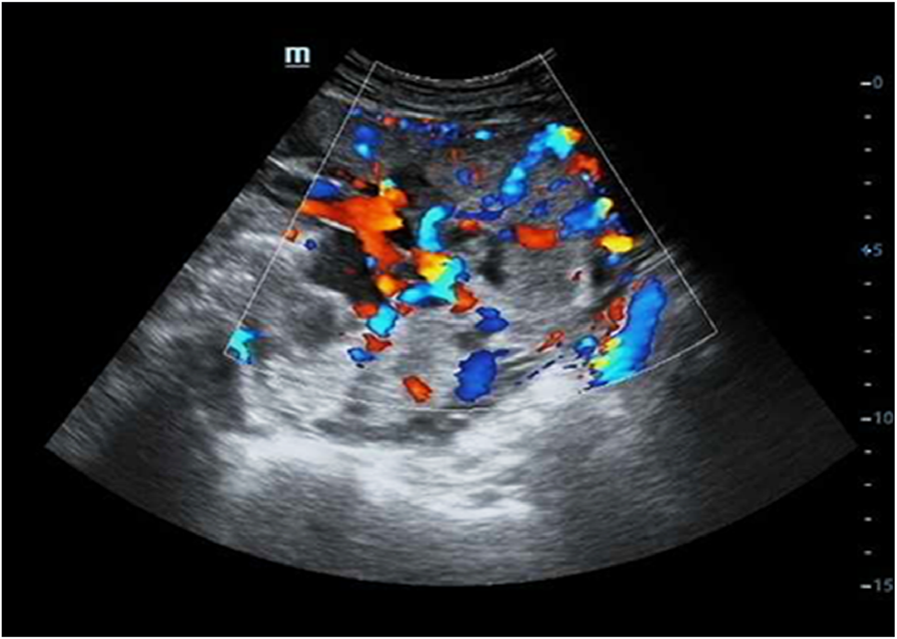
Ultrasound (US) imaging at gestational week 37 reveals total placenta previa with multiple vascular lacunae within the placenta. The placenta in the anterior wall reaches the serous layer of the uterus, and thinning of the uterine serosa-bladder wall interface is observed. Power doppler showing increased vascularity
